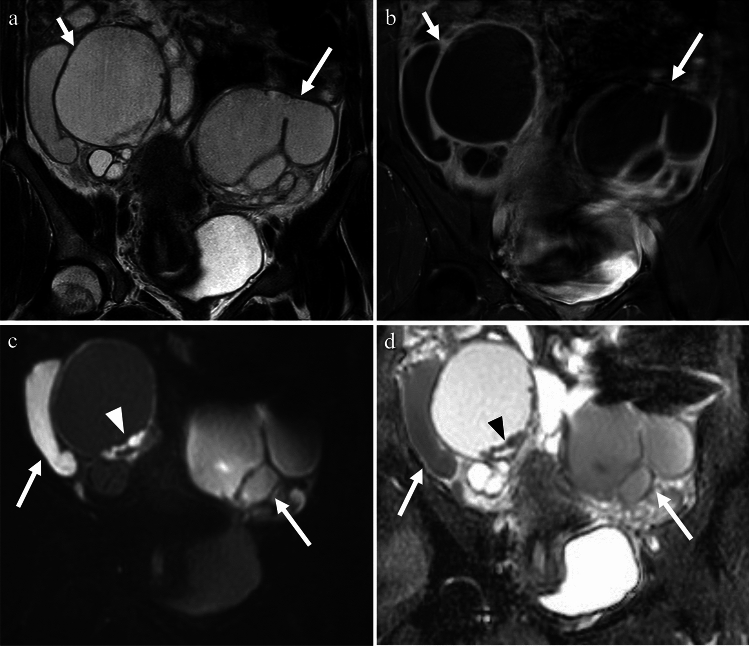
Woman in her 20 s with fever and lower quadrant pain who tested positive for Chlamydia. a T2-weighted image shows cystic lesions in bilateral adnexa (arrows), and tubular structures and plica indicating fallopian tubes. The contents of the fluid have a lower signal intensity than urine. b Thickening and enhancement of the cyst wall (arrows) seen on fat-saturated contrast-enhanced T1-weighted image are indicative of tubal empyema. c, d Some of the contents show a prominent high signal on DWI with decreased ADC (arrows), indicating pus. Debris with strong diffusion restriction is also observed (arrowheads)
